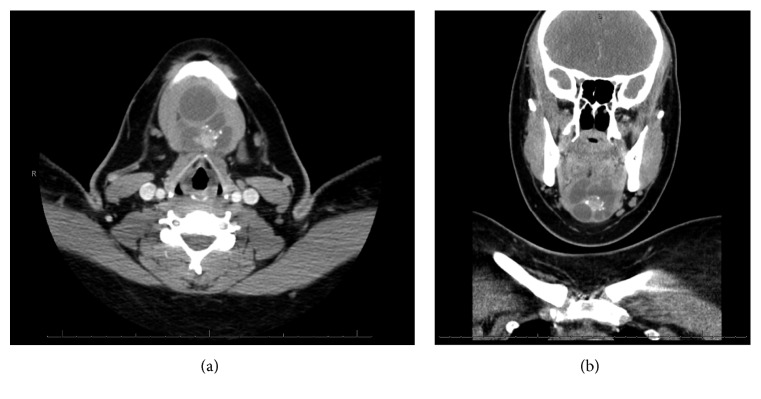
Axial (a) and coronal (b) computed tomography (CT) scan images of submental neck mass.
